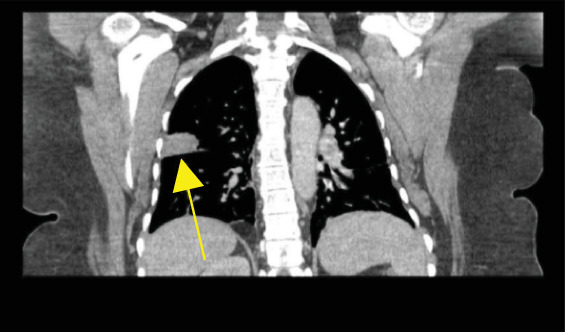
CT of the chest showing subpleural pulmonary opacity in the upper right lobe measuring 4.0 × 3.4 cm. Yellow arrow highlighting the right lung mass, biopsy proven MALT lymphoma.
